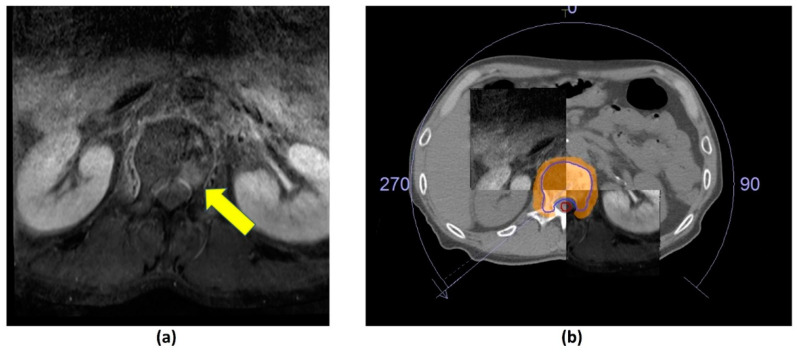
Axial post-contrast fat-suppressed T1-weighted MRI. (a) Metastatic lesion in the L1 vertebral body (yellow arrow). (b) CT and MR fusion for stereotactic body radiotherapy (SBRT) planning; 27 Gy over 3 fractions delivered using volumetric modulated arc therapy. Clinical target volume (CTV—blue outline), planning organ at risk volume (PRV, cord—red outline), 95% isodose (orange colour wash).
